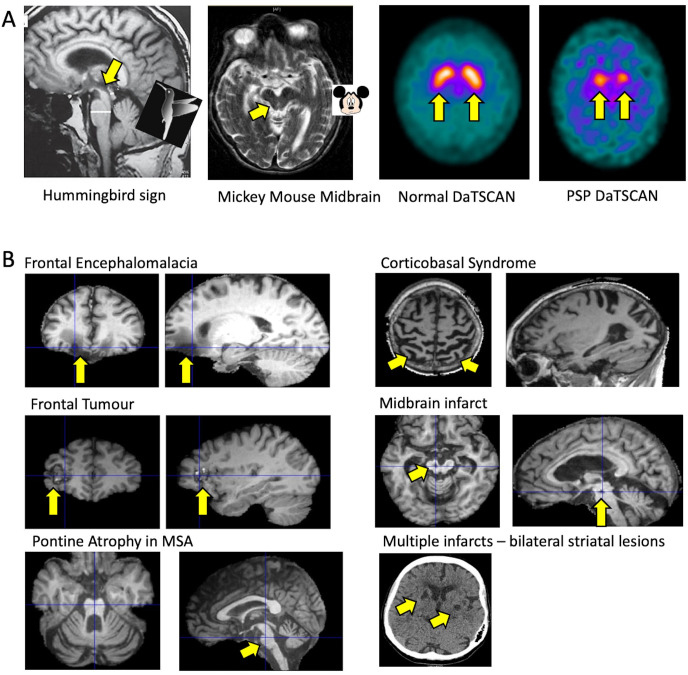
MRI and DaTscan features in PSP (A) and examples of MRI features of alternative diagnoses for PSP-like presentations (B), with arrows highlighling the salient feature or anomaly. MSA, multiple system atrophy; PSP, progressive supranuclear palsy.
